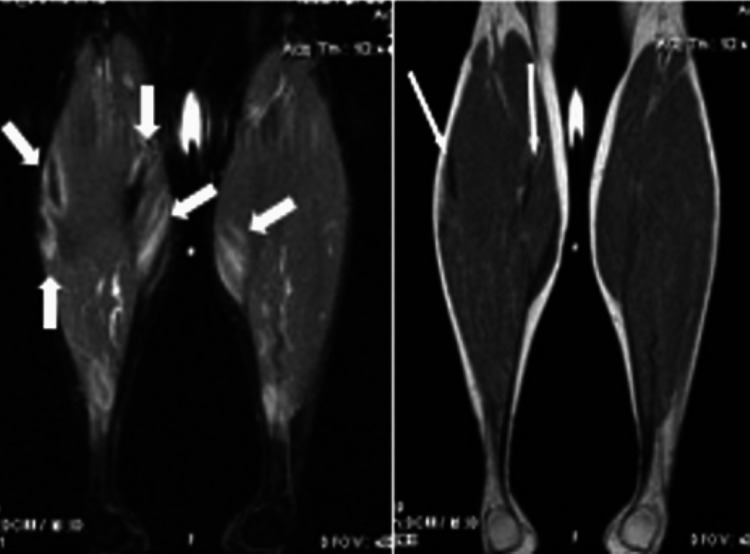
Magnetic resonance imaging (MRI) of both calves prior to therapy Coronal T2 short-tau inversion recovery (STIR) image of both calves, on the left, demonstrating increased signal within the gastrocnemius muscles bilaterally oriented along the muscle fibers. Some areas have central low signal. Corresponding coronal T1-weighted images, on the right, demonstrating only the central low signal areas.
